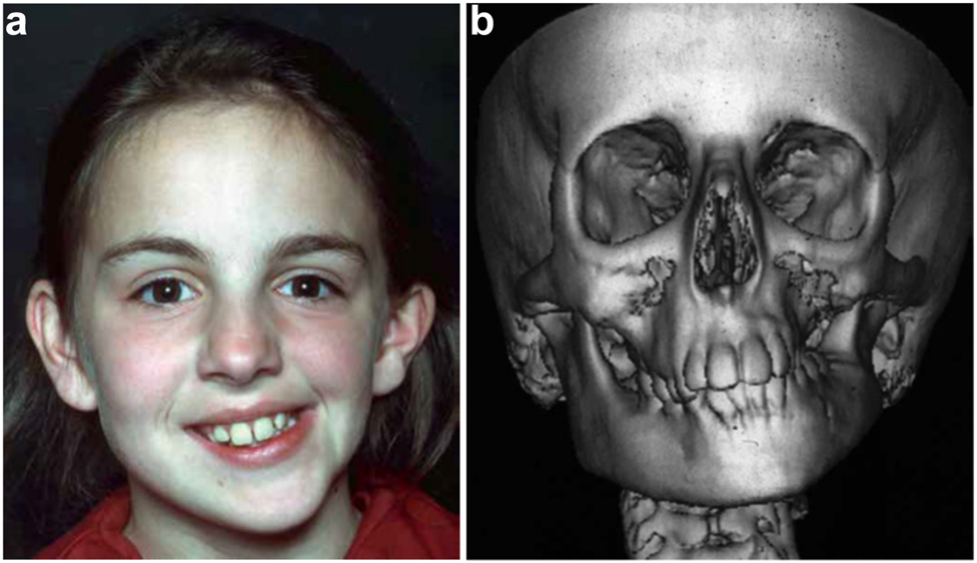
Clinical and CT example of Class II classification of HFM. (a) and (b) Example of Pruzansky IIa HFM aged 12 yrs.
